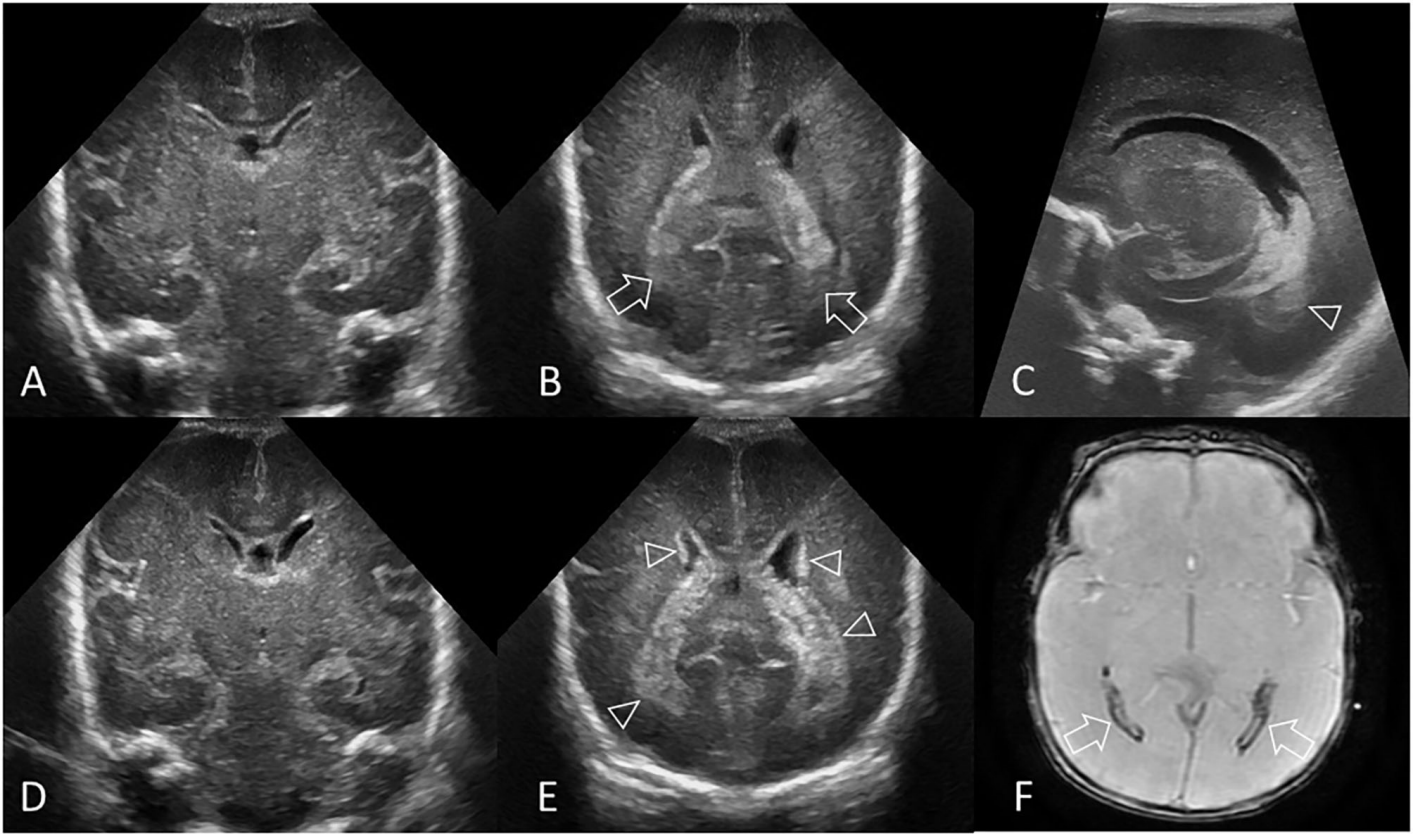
Hyperechogenic ependyma as indirect sign of IVH. Ultrasound and MRI scan in a preterm infant (GA 24 weeks 3 days). (A) Coronal scan at the level of the frontal horns of the lateral ventricles shows no evidence of GMH, IVH, or ventricular enlargement. (B) Coronal scan at the level of the trigone of the lateral ventricles shows a small volume of echogenic clot within the posterior body of both ventricles (arrows); this finding was not recognized at time of original reporting. (C) Parasagittal scan through the left lateral ventricle also shows the small volume IVH (arrowhead). (D) Follow up coronal scan 14 days after birth at the level of the frontal horns still does not show clear evidence of germinal matrix clot. (E) Coronal scan at the level of the trigone of the lateral ventricles shows new hyperechogenic ependyma involving both lateral ventricles (arrowheads), with more extensive IVH within the bodies of the lateral ventricles. No hydrocephalus has developed. The presence of this hyperechoic ependyma on the follow-up scan facilitated a retrospective diagnosis of grade II GMH-IVH in this infant. (F) Axial T2^*^ gradient-echo MR image 3 months after birth shows increased susceptibility effect (hypointense signal) that outlines the occipital horns of both lateral ventricles (arrows) from hemosiderin/ferritin and confirms presence of earlier IVH. Several microhemorrhages were also identified in the bilateral germinal matrix regions on this blood sensitive MR sequence (not shown).
